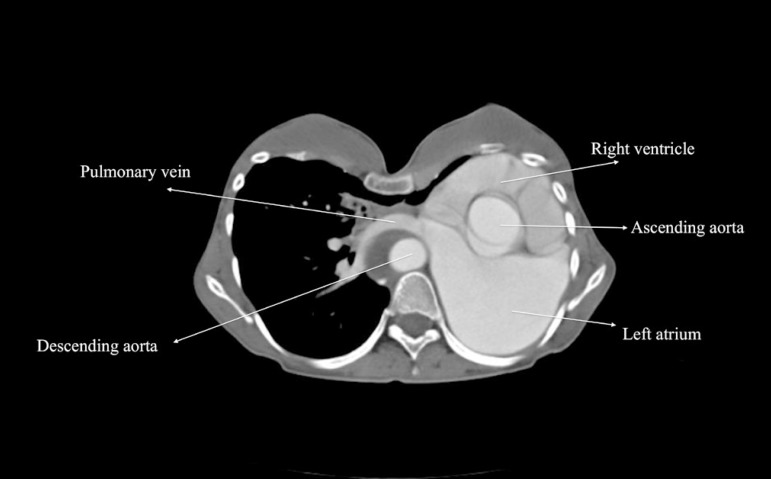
Contrast-enhanced computed tomography scan.
